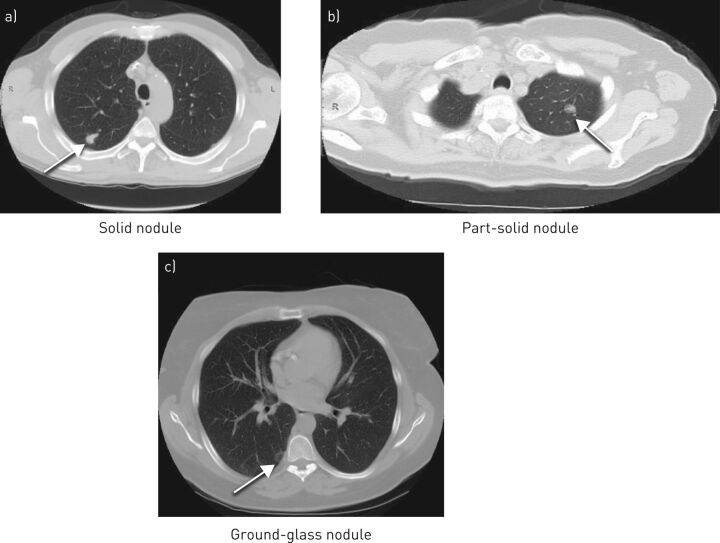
Computed tomography images of different types of solitary pulmonary nodules.
